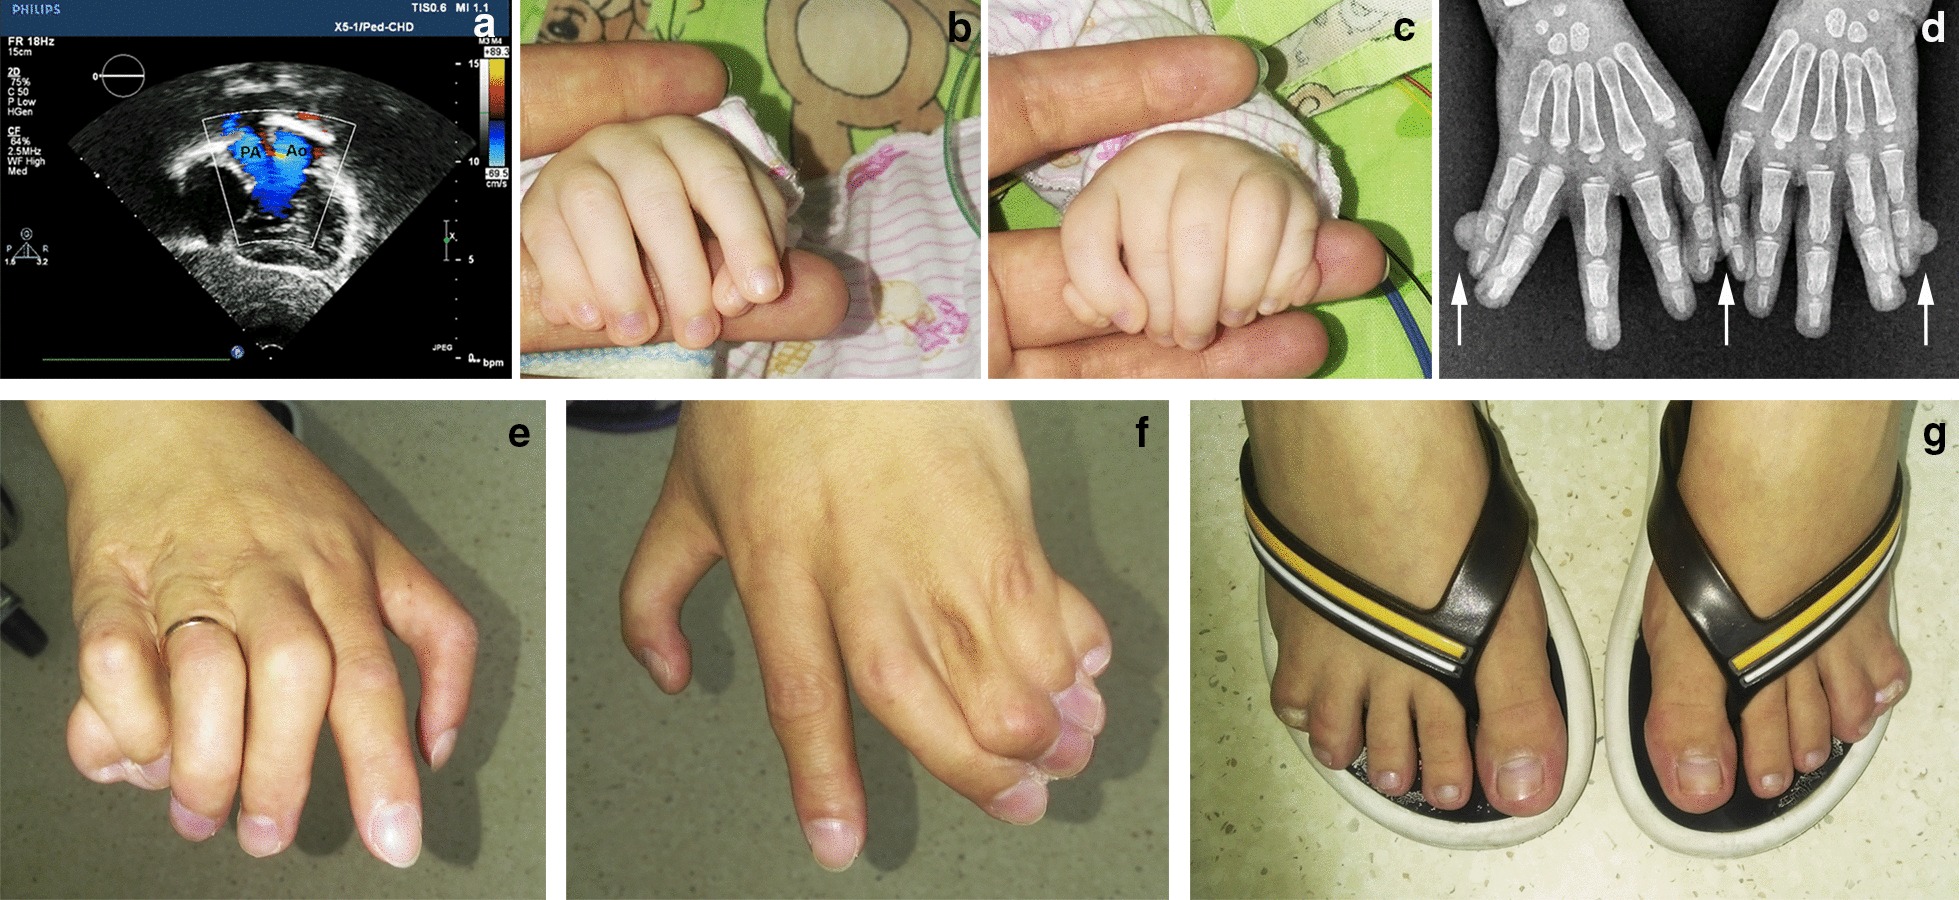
Clinical features. a Echocardiogram of the patient indicating double outlet right ventricle. PA—pulmonary artery, Ao—aorta. b–d Right and left hands of the proband with TPT-PS. Note bilateral triphalangeal thumb with asymmetrical pre- and post-axial polydactyly (arrows) and skin syndactyly involving fingers IV and V. e–g Hands and feet of the proband`s mother. Note triphalangeal thumb, post-axial polydactyly with total skin syndactyly of fingers III-V as well as syndactyly of fingers IV/V on the feet
